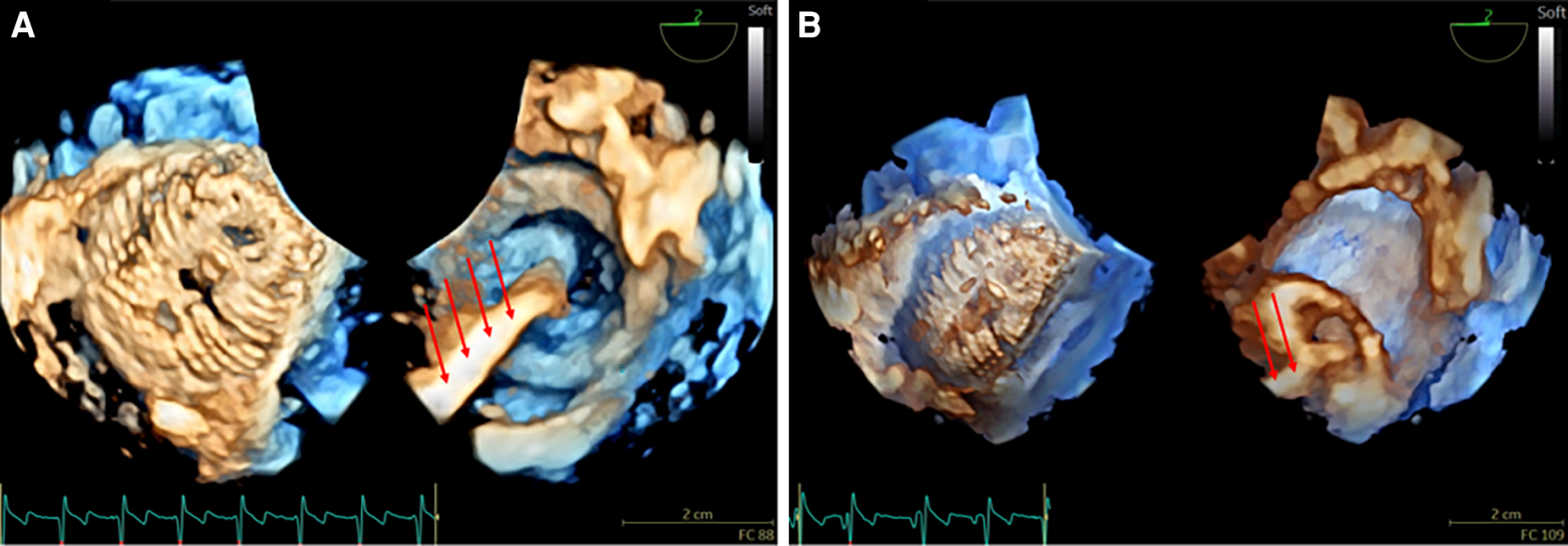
3D real-time TOE of the atrial septal device before release in a 17 kg patient with a central atrial septal defect (ASD). (A) The figure demonstrates a 3D en face view of a 22 mm device from both the left atrium (left panel) and the right atrium (right panel) with the wire still attached (red arrows) (Supplementary Video S1). (B) Depiction of the “Minnesota maneuver” showing the pull on the right disk (Supplementary Video S2). 3D: three-dimensional; ASD, atrial septal defect; LA, left atrium; RA, right atrium; TOE, transoesophageal echocardiography.
